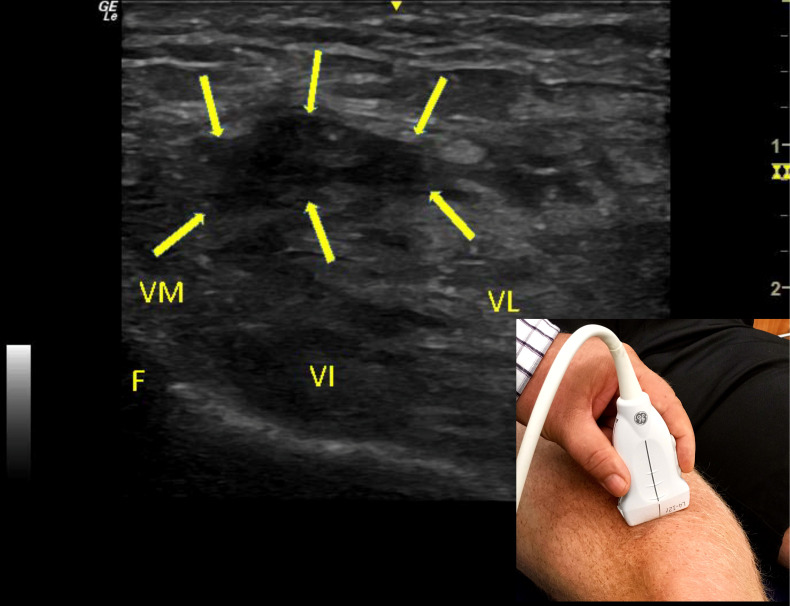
Post-injury short-axis ultrasound image of the Rectus Femoris. Disruption of the musculotendinous fibers of the rectus femoris can be visualized. A large hypoechoic gap in the rectus femoris is present, denoting the presence of significant fluid (arrows). Deeper areas of hypoechoic signaling indicating straining of the vastus medialis and lateralis and intermedius. Positioning of the ultrasound probe is seen in the bottom right-hand corner of the image. Abbreviations: F: femur; VL: vastus lateralis; VM: vastus medialis; VI vastus intermedius.
